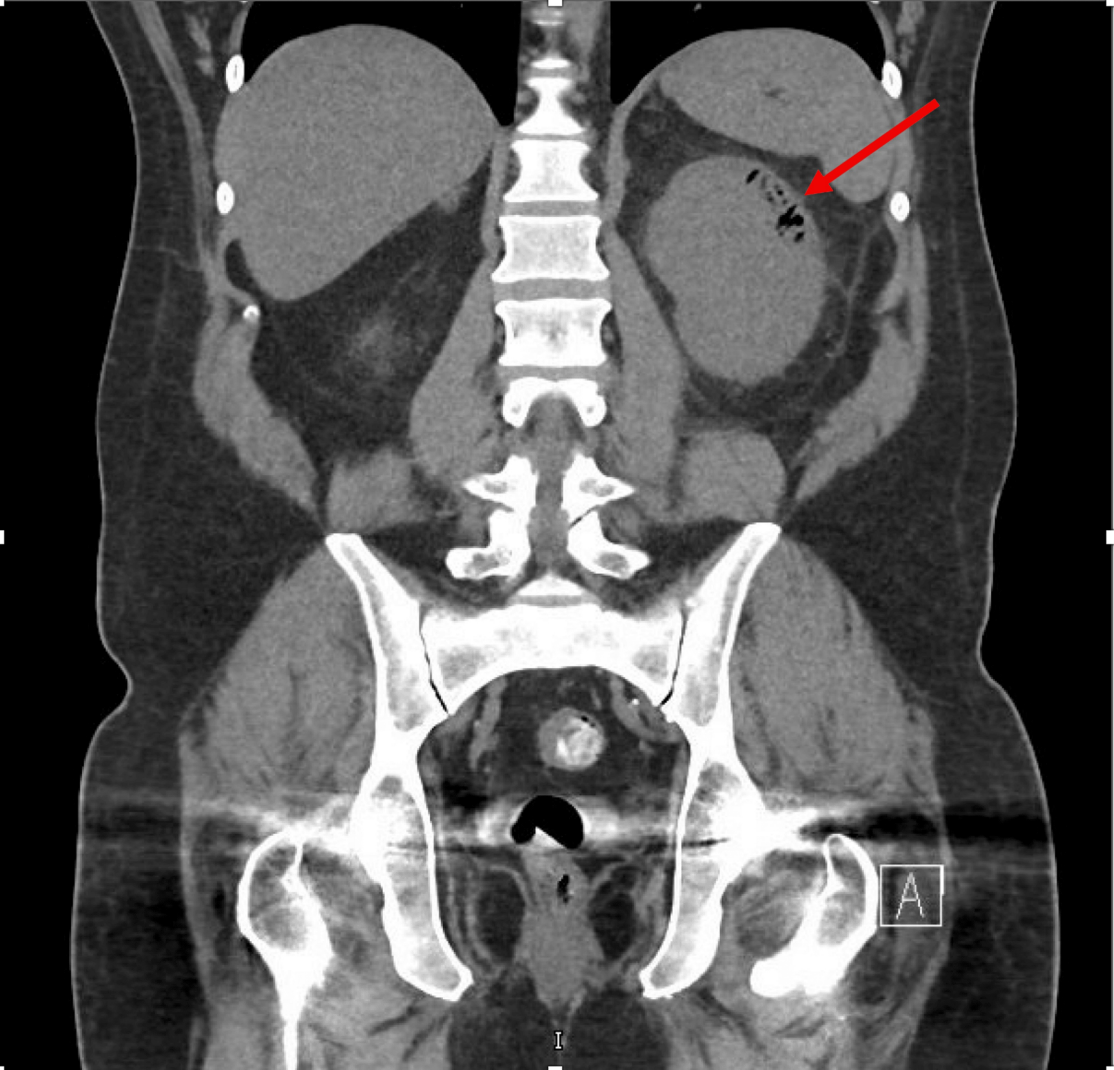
Left emphysematous pyelonephritis found on CT-guided biopsy
